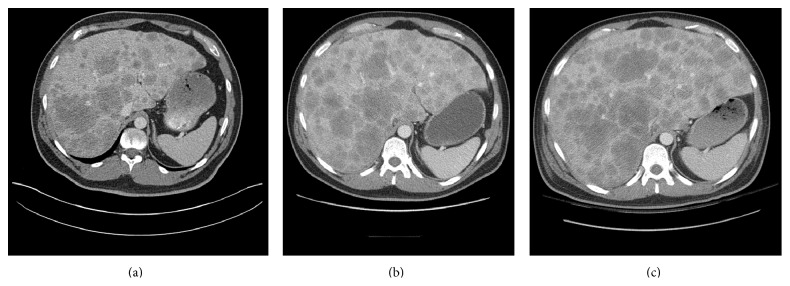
Case 1: CT scan of the abdomen showing rapid progression in both size and number of the previously noted innumerable liver metastases in a patient with mCRC: (a) day 0, (b) day 10, and (c) day 18.
